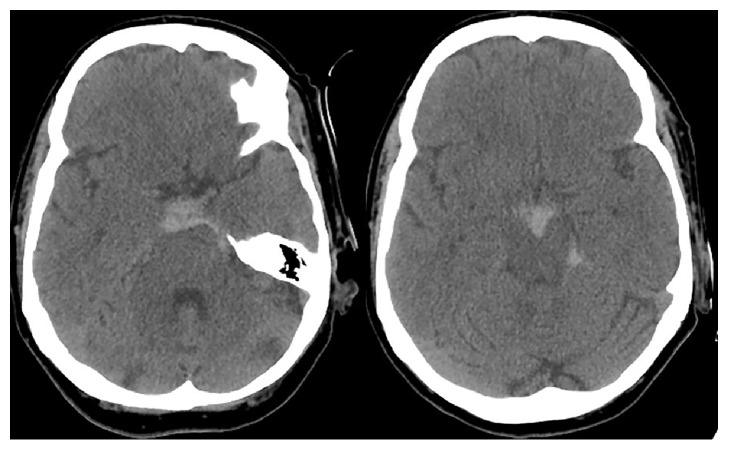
CT of the head demonstrated subarachnoid hemorrhage in the perimesencephalic, prepontine, interpeduncular cisterns.
